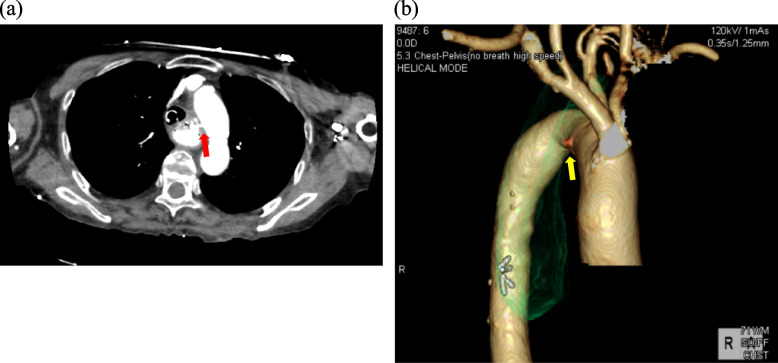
Computed tomography (CT) angiogram showing a fistula between the gastric conduit and the thoracic aorta. a Large amount of blood clots occupying the gastric conduit (red arrow). b Arterial bleeding from the anterior wall of the gastric conduit (yellow arrow)
